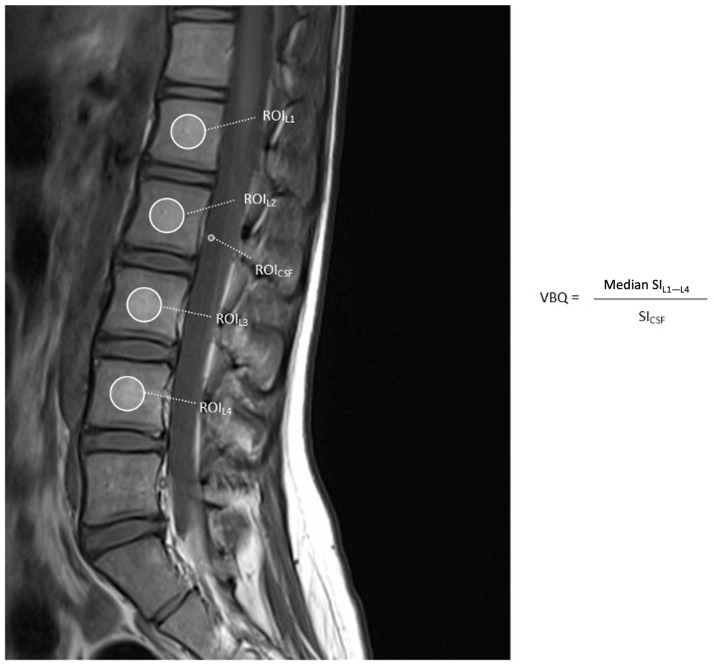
Non-contrast-enhanced T1-weighted MRI of the lumbar spine showing regions of interest (ROIs) used to calculate conventional L1–L4 VBQ score. SI = signal intensity; CSF = cerebrospinal fluid.
